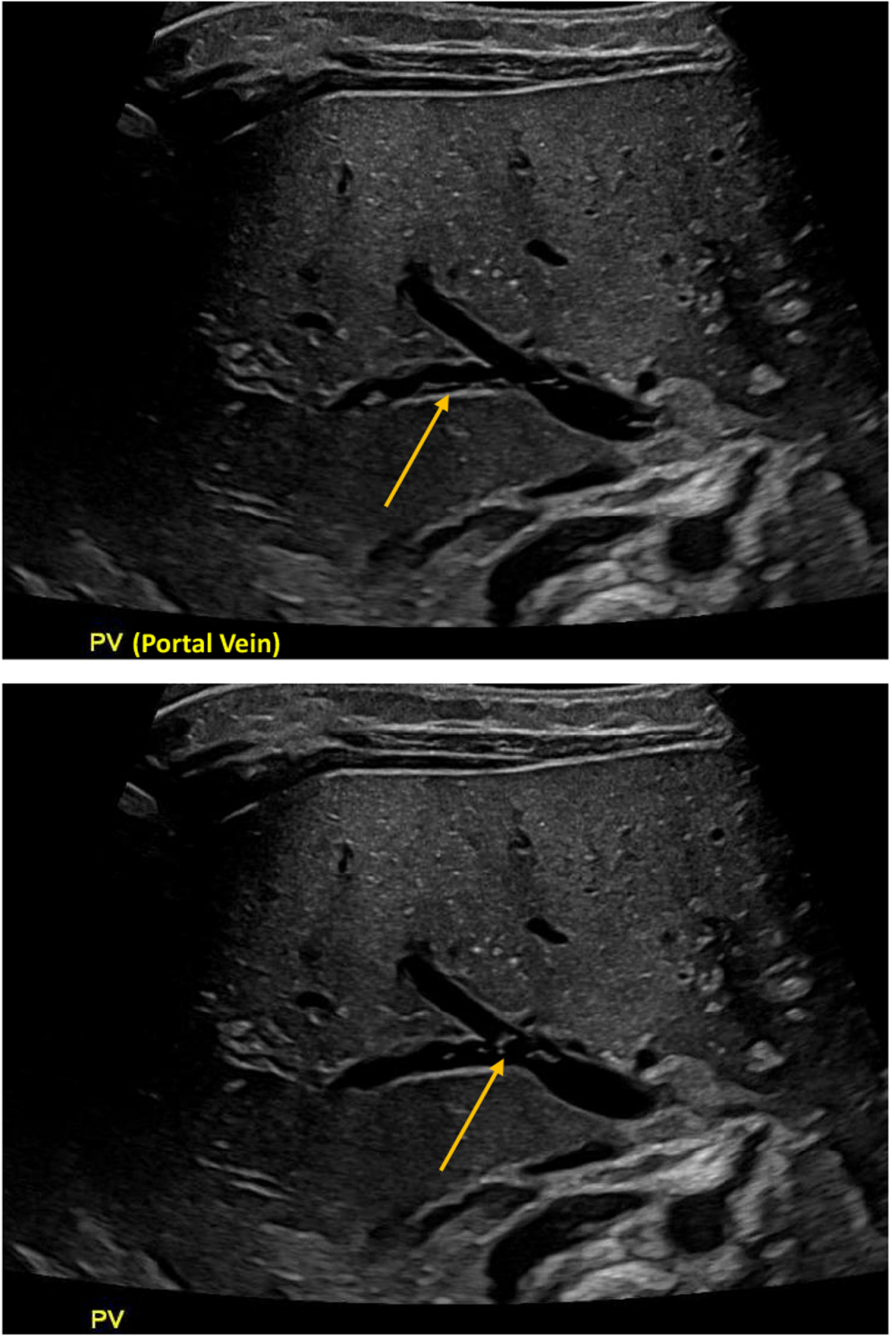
Two right upper quadrant ultrasound images showing multiple dynamic echogenic foci in the portal vein representing portal venous gas.
